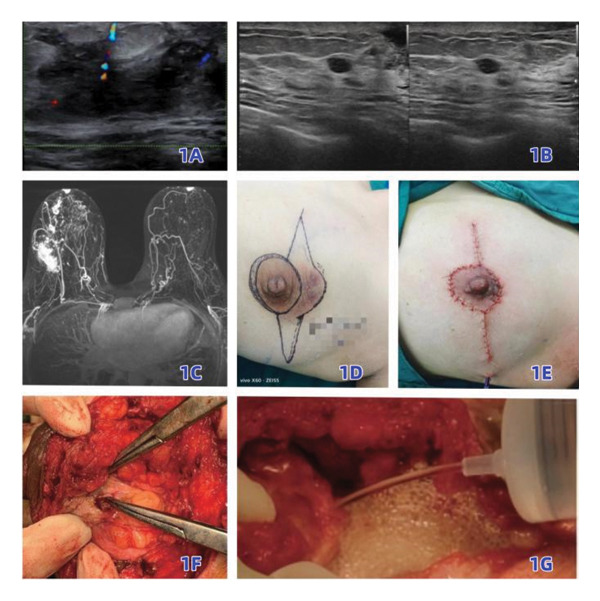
Images related to ultrasound‐guided precise lesion resection + primary oncoplastic reconstruction: preoperative ultrasound examination for lesion localization (1A); intraoperative ultrasound repositioning of tiny lesions (1B); preoperative breast MRI (1C); preoperative breast lesion (1D); primary oncoplastic reconstruction + postoperative drainage (1E); curette removal of tiny lesions and precise resection of necrotic tissue (1F); intraoperative irrigation (1G).
